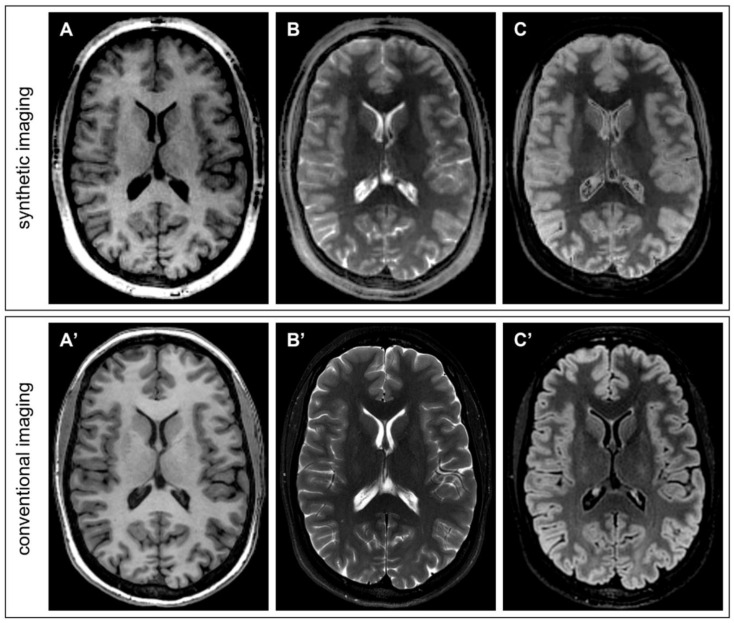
Direct qualitative comparison between synthetic images obtained with PySynthMRI from QTI-derived maps (top row) and conventional T1-weighted (A,A’), T2-weighted (B,B’) and T2-FLAIR (C,C’) images of conventional acquisitions (bottom row).
